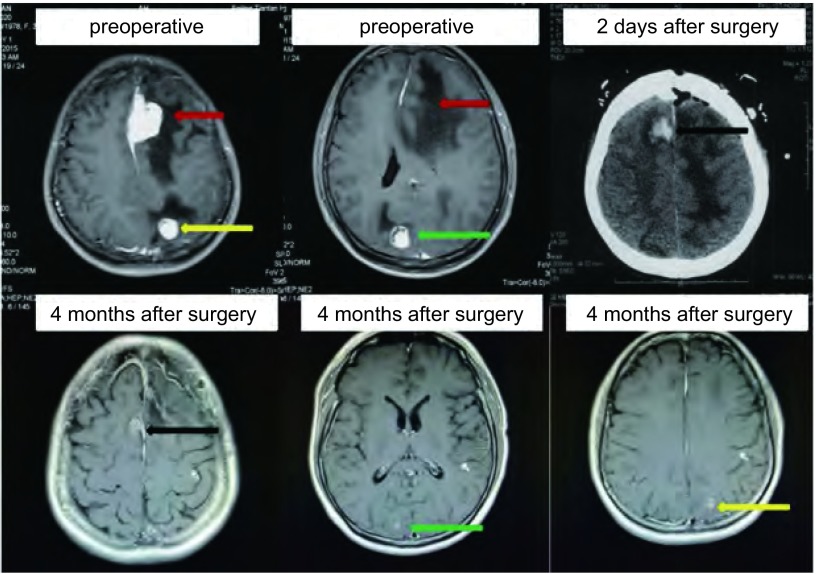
患者治疗前后的头MRI。红箭头:左额叶病灶; 黄箭头:左顶叶转移灶; 绿箭头:右枕叶转移灶; 黑箭头:右额叶出血灶, 术后2天出现。 Magnetic resonance imaging (MRI) of brain before and after treatment.Red arrow:metastatic lesions of left frontal lobe; yellow arrow:metastatic lesions of left parietal lobe; green arrow:metastatic lesions of right occipital lobe; black arrow:hemorrhagic lesion of left parietal lobe occurred 2 days after surgery.
